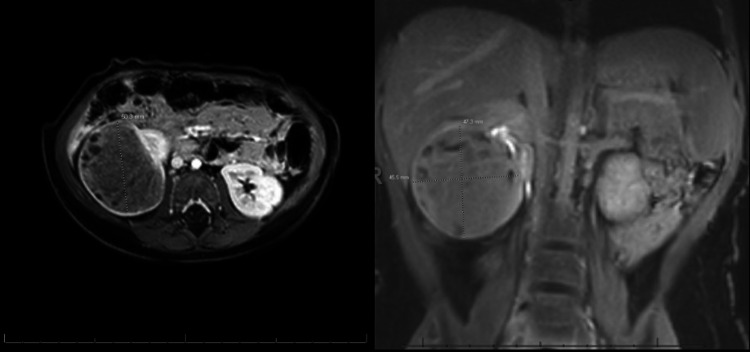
MRI revealing well-defined, predominantly solid hypoenhancing right renal mass with a small cystic area in the periphery of the lesion
